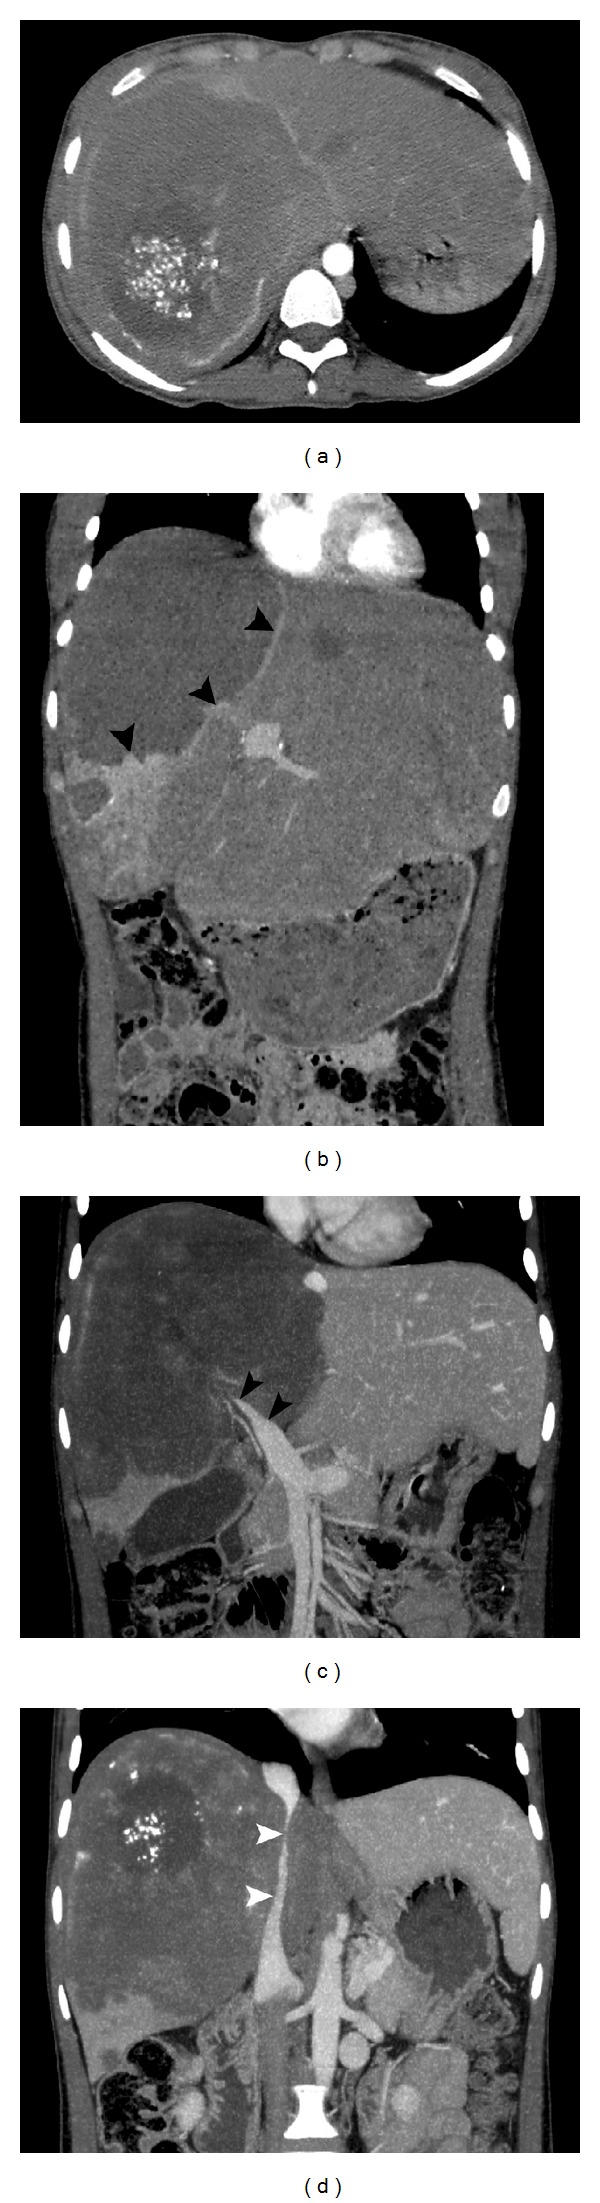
Axial enhanced abdominal CT image (a) demonstrates an infiltrative tumor-like hepatic lesion with irregular margins and heterogeneous contents, including amorphous calcifications. The fibroinflammatory tissue surrounding the mass (arrowheads) exhibits mild contrast enhancement in a delayed phase coronal reformatted CT image (b). Right portal vein branch thrombosis (arrowheads) is shown in acoronal contrast-enhanced maximum intensity projection (MIP) image (c). Another coronal MIP image (d) depicts severe narrowing of the intrahepatic vena cava inferior (arrowheads).
